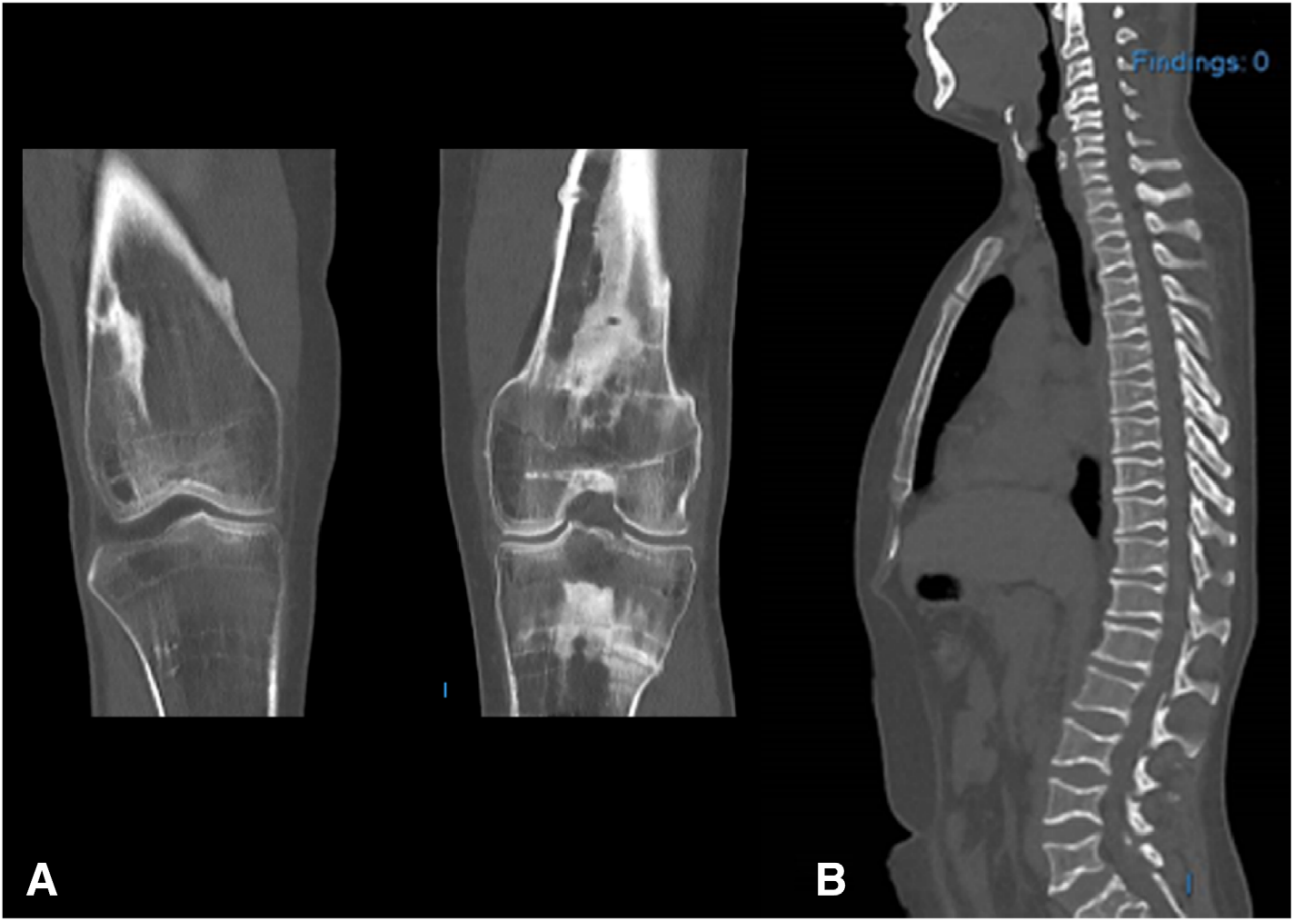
Typical radiologic characteristics fitting with osteosclerotic metaphyseal dysplasia of femur/tibiae (A) and vertebrae (B). (A) Coronal CT image of both knees showing bilateral, but predominantly on the left knee, sclerotic bands in the metaphysis of the femur and tibia in transverse and longitudinal direction. This resembles an osteopathia striata, but largely located in the metaphysis. (B) Sagittal CT image of the spine shows biconcave loss of height of the vertebrae with a fish‐like appearance. Some vertebrae also show more sclerotic endplate changes.
